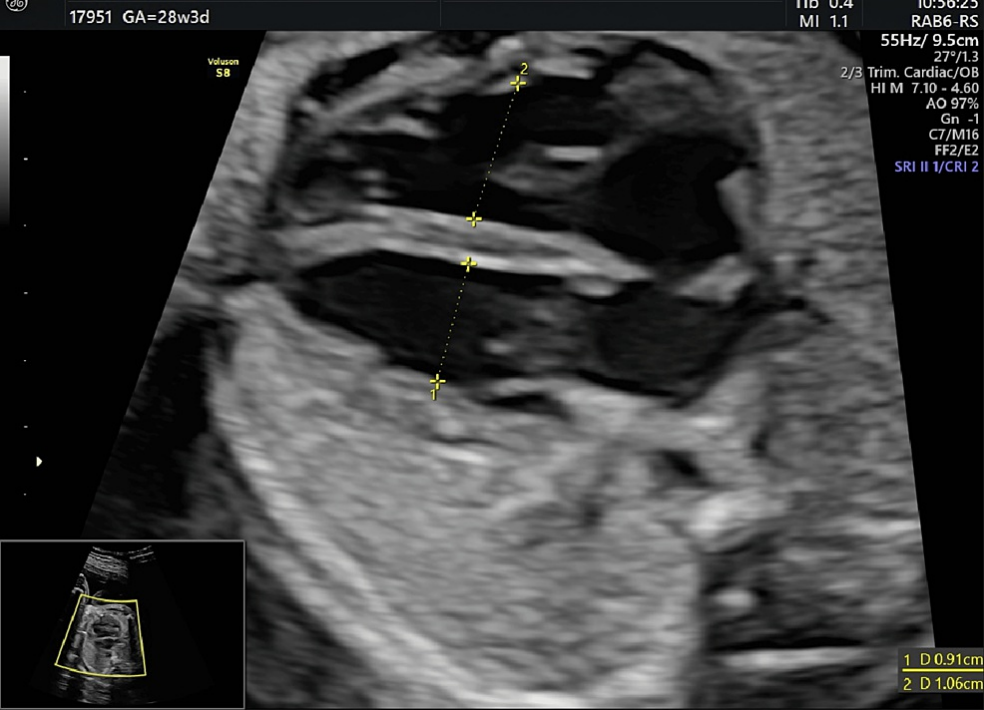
Detailed fetal echocardiography demonstrating the mildly dilated ventricles of the fetal heart
